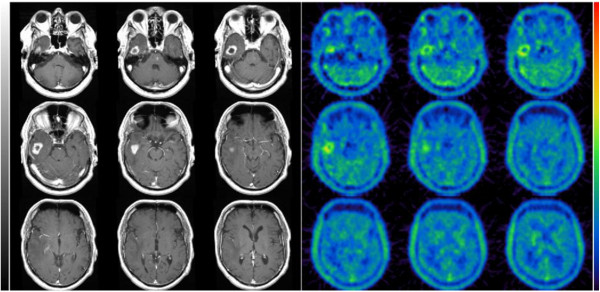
Example of recurrent brain metastasis: the study shows pathologic contrast enhancement on T1-weighted MRI and corresponding increased [^18^ F]-FET uptake. While radiation necrosis was suspected in MRI, PET showed a pathologic FET uptake as seen in recurrent metastasis.
